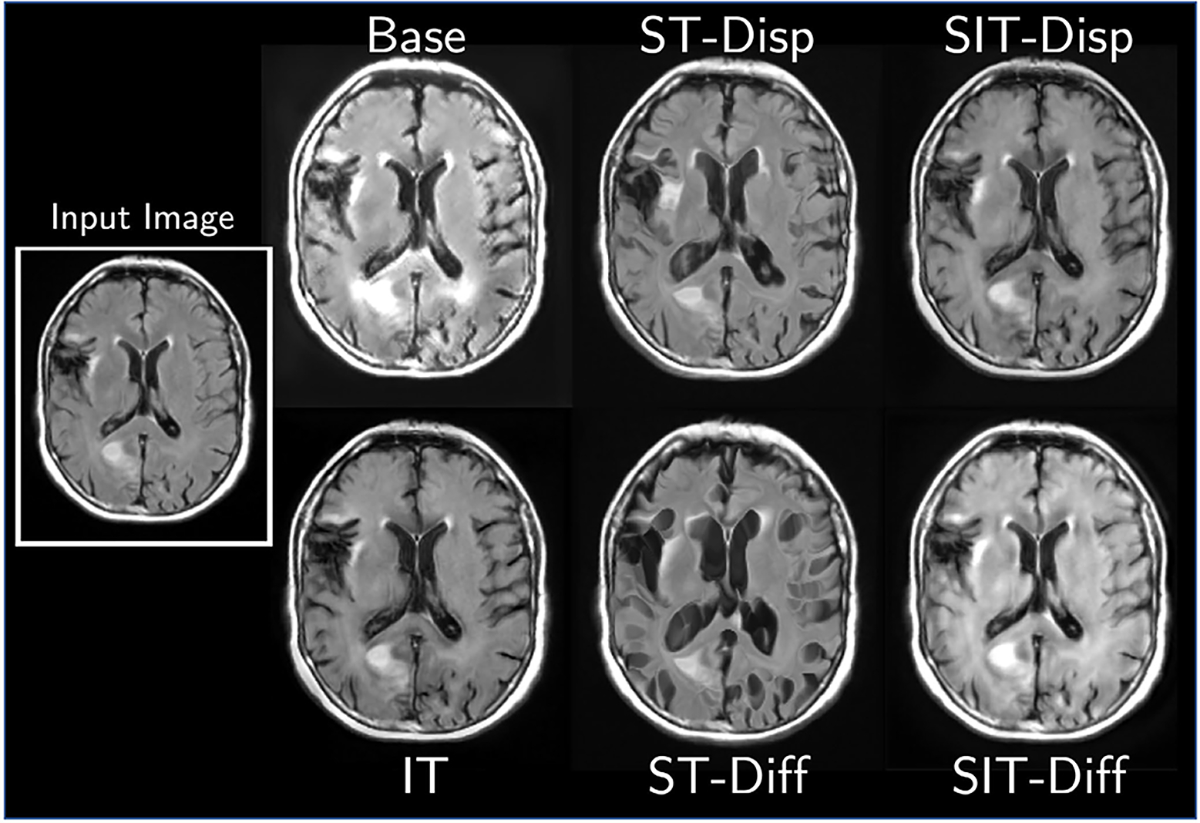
A scan from MRI-GENIE translated to a different age (originally 59 years old, translated to 84 years old) using different parameterizations of the generator in StarGAN.
